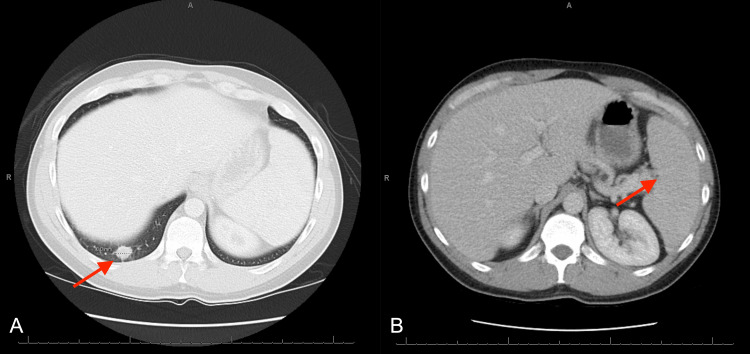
CT chest and abdomen with IV contrast (A) Lung nodule in the right lower lobe measuring 1.6 cm decreased from prior CT. (B) Decrease in diffuse splenic parenchymal heterogeneity compared to prior CT.
